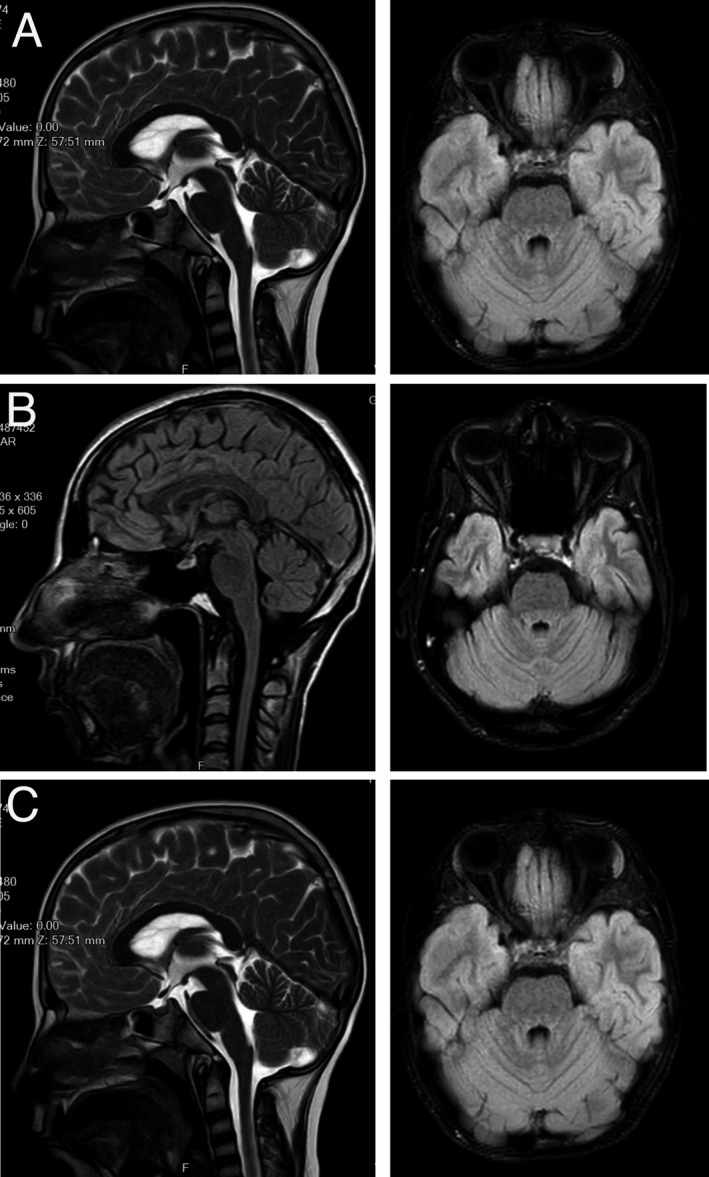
Sagittal T1‐weighted MRI of brain. Axial and sagittal Fluid‐attenuated inversion recovery (FLAIR) brain MRI images of Cases (A) IV:10, (B) IV:11, and (C) V:1 reveal normal cerebellum and indicate no abnormality.
